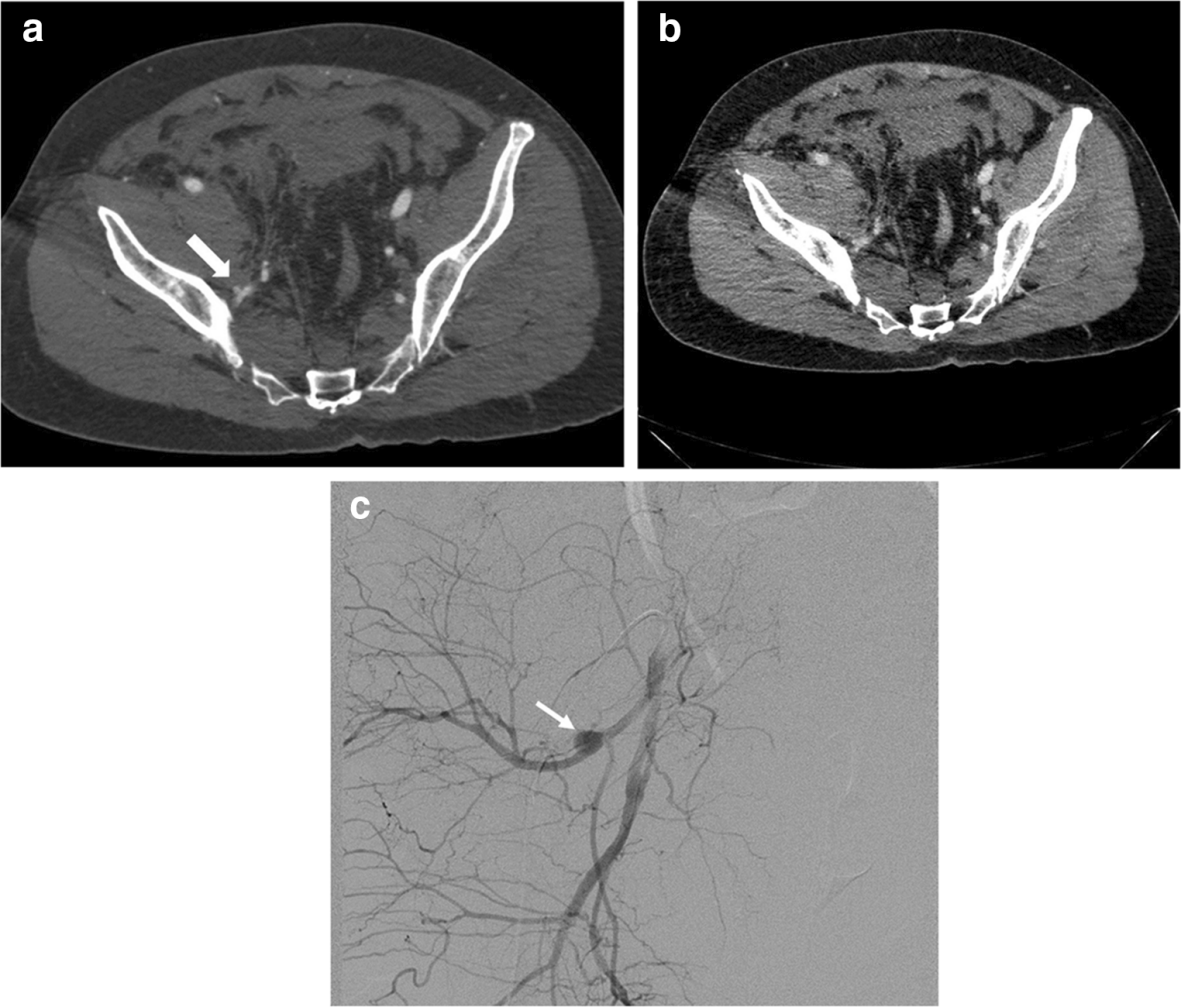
Pseudoaneurysm of the left superior gluteal artery visible on arterial phase CT ((a), white arrow), but not on portal venous phase CT (b). Corresponding angiography image ((c), white arrow)
